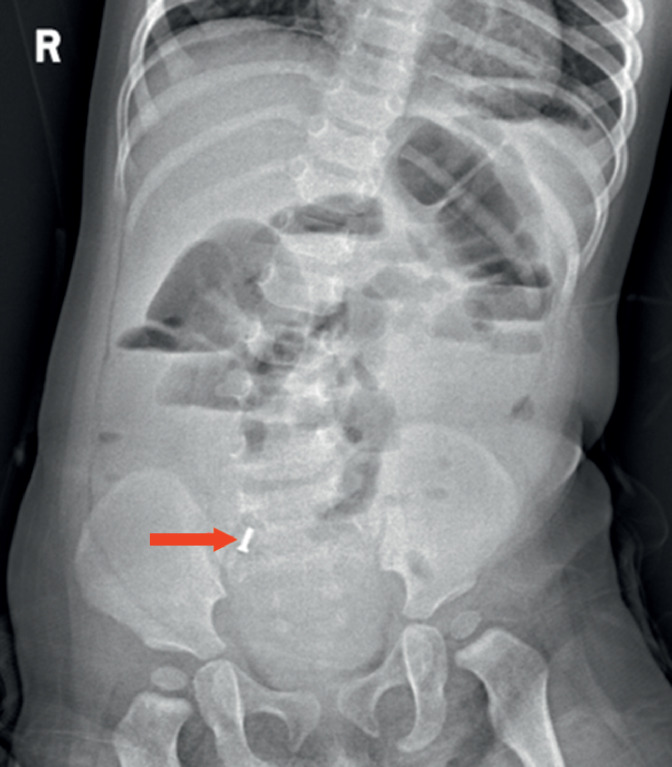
X-ray showed a screw (arrow) in the right lower quadrant in a 13-month-old girl.
